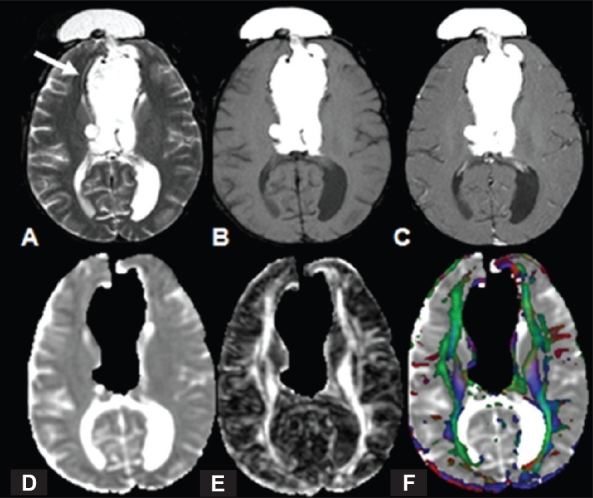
A 25-year-old woman with agenesis of the corpus callosum and an interhemispheric lipoma with subcutaneous extension. MRI shows an interhemispheric hyperintense mass protruding into the subcutaneous tissue (arrow) on T2W (A) and T1W (B) images, with no enhancement on the post-contrast T1W image (C). MD map (D) generated from the fat-suppressed DTI data, shows hypointensity in the lesion consistent with fat. FA (E) and color-coded FA fused with MD map (F) show agenesis of the corpus callosum
